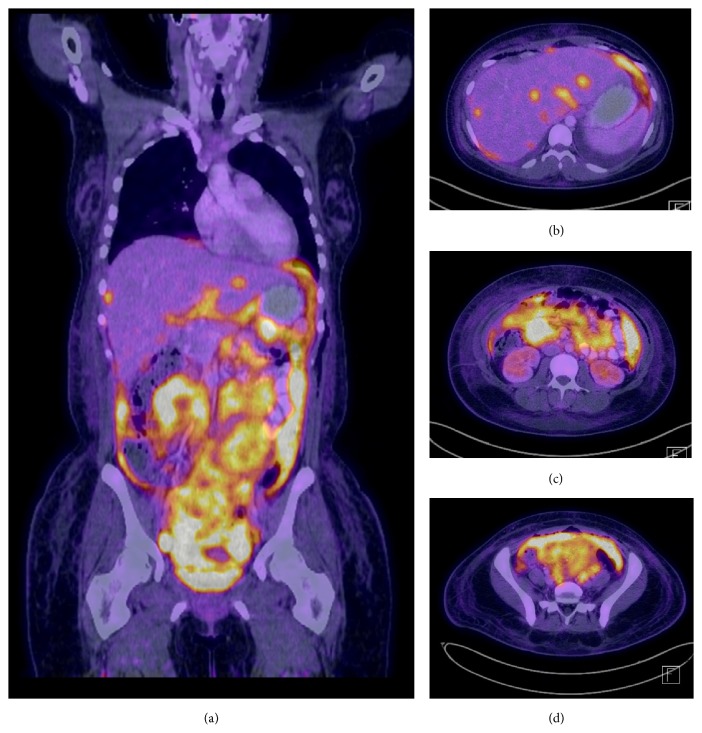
PET/CT imaging of the body performed in June 2017. (a) Coronal Body. (b) Axial upper abdomen. (c) Axial mid abdomen. (d) Axial low abdomen. Findings of diffuse and permeative abnormal hypermetabolic activity throughout the cavity corresponding to thickening and caking of the omentum and small pelvic free fluid. Hypermetabolic activity corresponding to multiple low attenuating liver lesions and retrosternal iliac chain nodes suspicious for malignancy.
